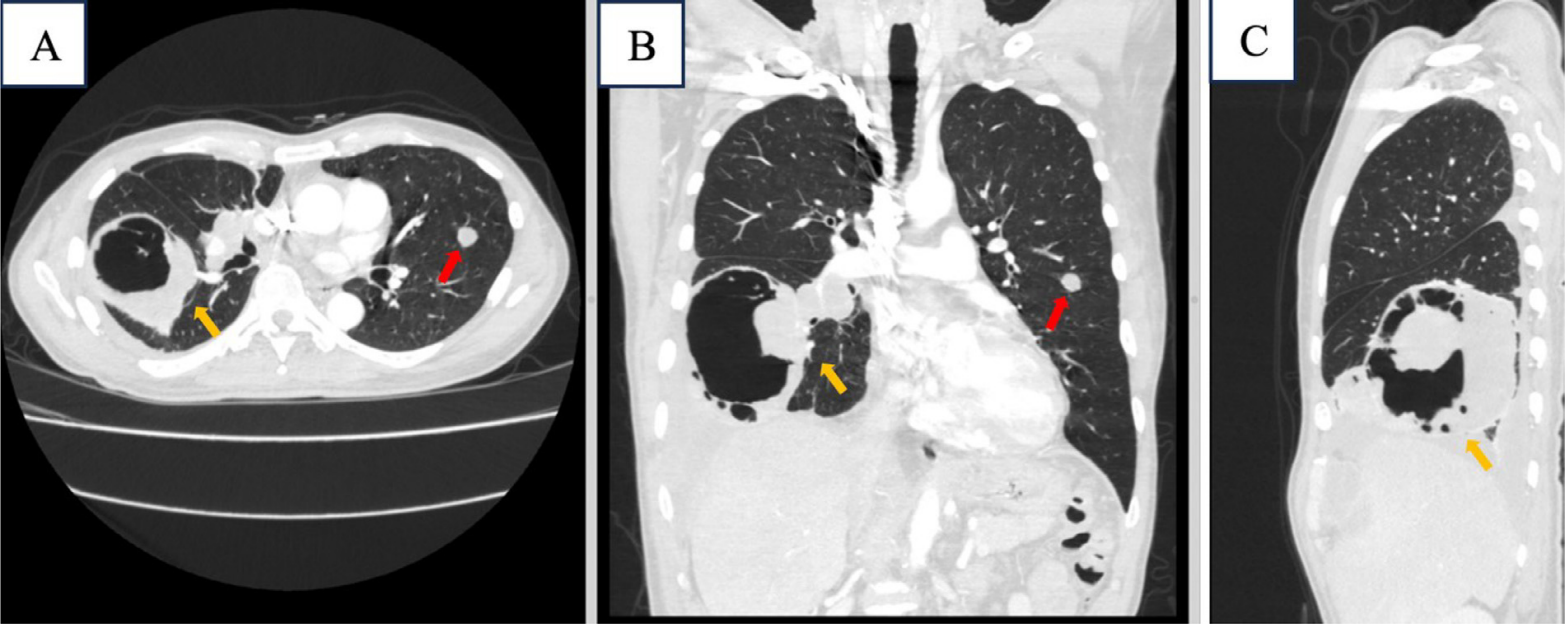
Contrast-enhanced CT-scan images of the chest region (A) Axial, (B) Coronal and (C) Sagittal. Show cavitating lesion with solid and fluid component forming an air-fluid level (yellow arrow) and nodule in superior lingula segment of the superior lobe of the left lung (red arrow).
